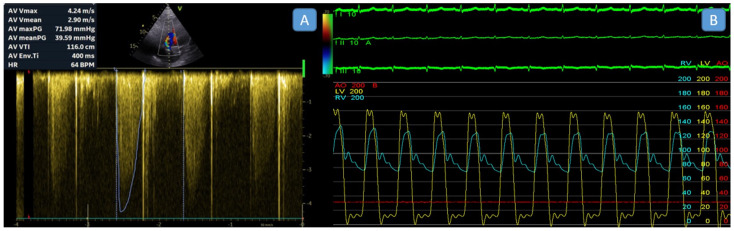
(A). Measurement of the gradient across the aortic mechanical valve by transthoracic echocardiography. (B). Simultaneous invasive measurement of aortic and left ventricular pressure gradients. The yellow line represents left ventricular pressure, and the blue line represents aortic pressure. In this patient, who had a surgical aortic valve replacement, transthoracic echocardiography indicated a pressure gradient of 59/38 mmHg across the aortic valve, while the invasive peak-to-peak gradient measurement was 19 mmHg.
